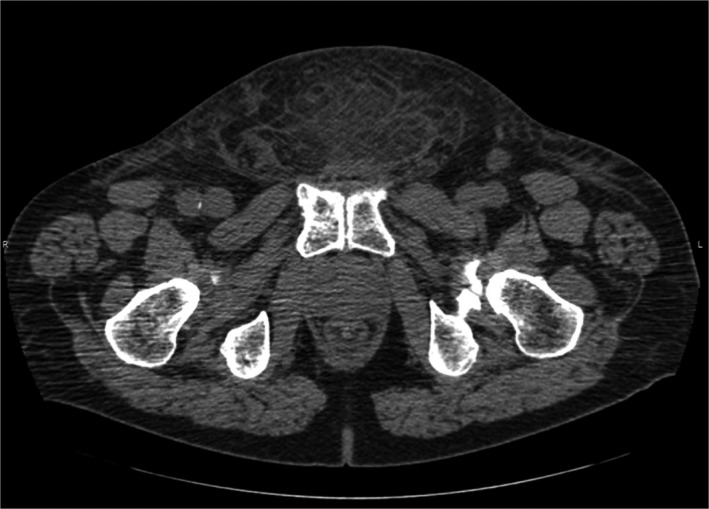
CT showing fat stranding in lower pelvic wall
